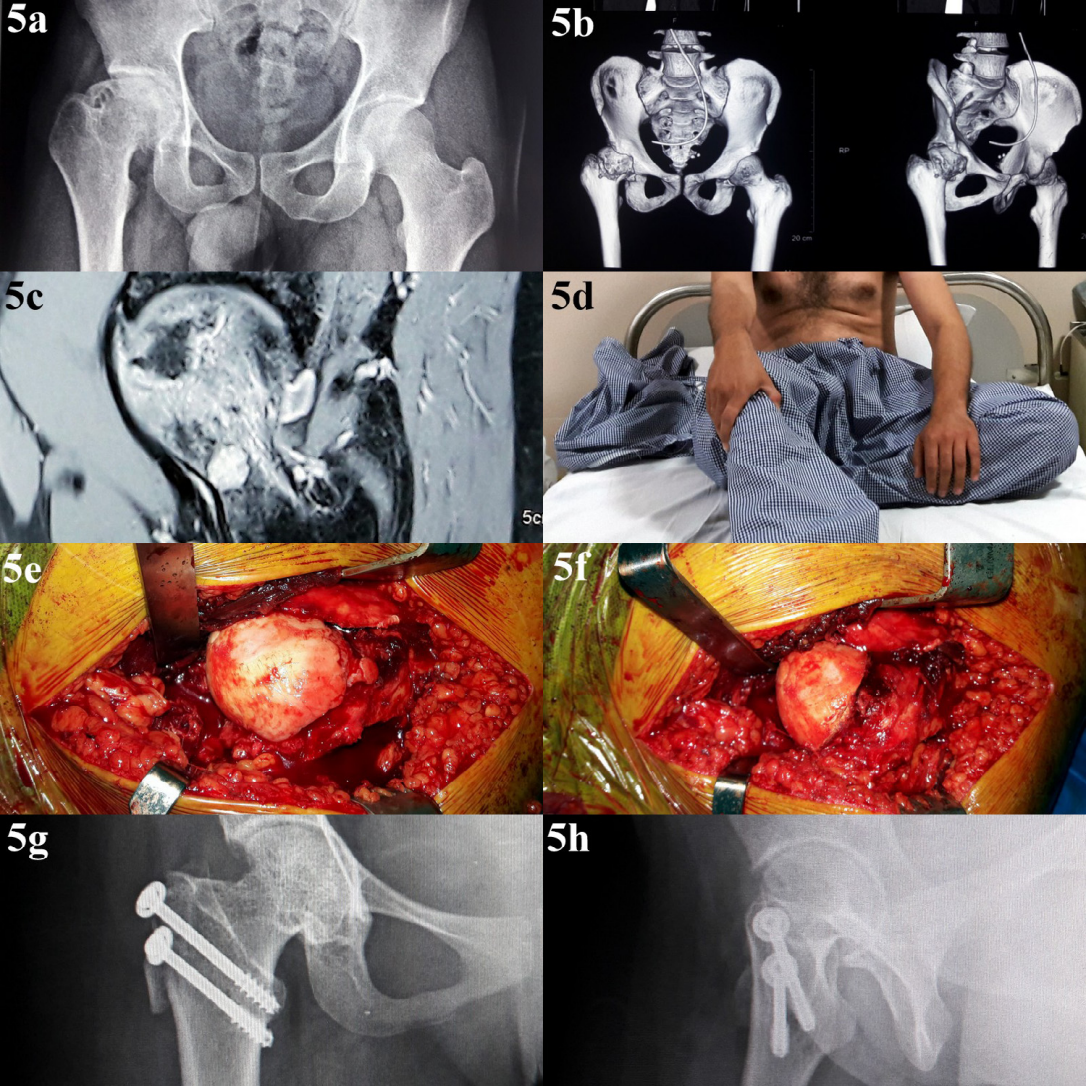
Follow-up of a 35-year-old male presenting with symptoms of hip impingement after steroid-induced ONFH; panel a: preoperative radiograph showing cam deformity in right femoral head; panel b: preoperative CT scan showing extent and location of cam deformity in the femoral head; panel c: MRI scan earlier in the course of disease-confirming changes of ONFH; panel d: clinical radiographs showing restricted hip motion due to cam deformity of femoral head; panel e: intraoperative picture showing cam deformity at femoral head–neck junction and preserved articular cartilage; panel f: intraoperative picture after femoral head osteochondroplasty; panels g and h: post-operative radiographs showing restoration of femoral head sphericity.
